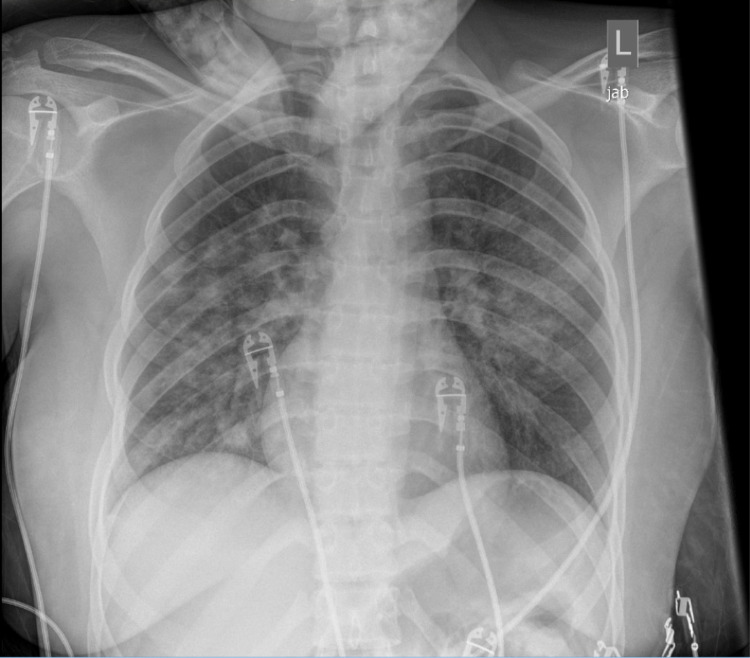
Anterior-posterior chest radiograph of the patient on post-operative day 1.
